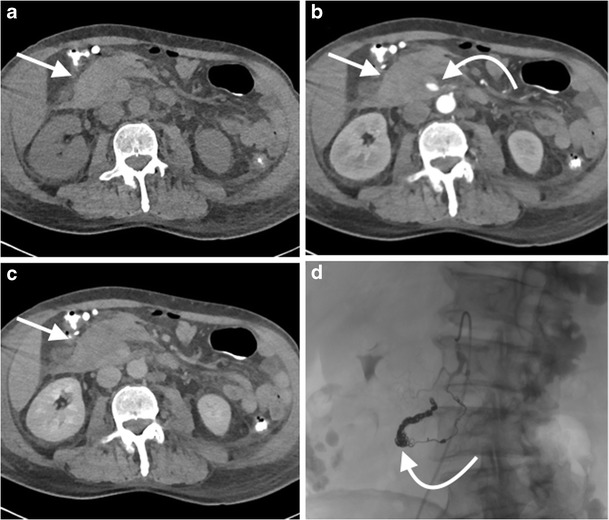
A 50-year-old man with oesophageal cancer was referred to CT following discovery of a mesenteric haematoma during exploratory laparoscopy. Axial non-contrast (a), arterial phase (b) and 70-s post contrast (c) images demonstrate an ill-defined mesenteric haematoma (*arrow*), a pancreaticoduodenal arcade pseudoaneurysm (*curved arrow*), with no active extravasation. The patient was taken to the interventional radiology suite and the pseudoaneurysm was occluded with coils; selected spot fluoroscopic image (d) demonstrates the occluded pancreaticoduodenal arcade filled with coils (*curved arrow*)
